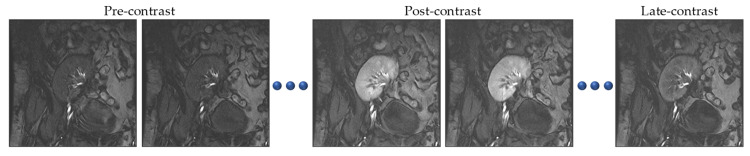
Sample DCE-MRI scans of one subject visually reflecting the effect of the injected contrast agent on the kidney.
